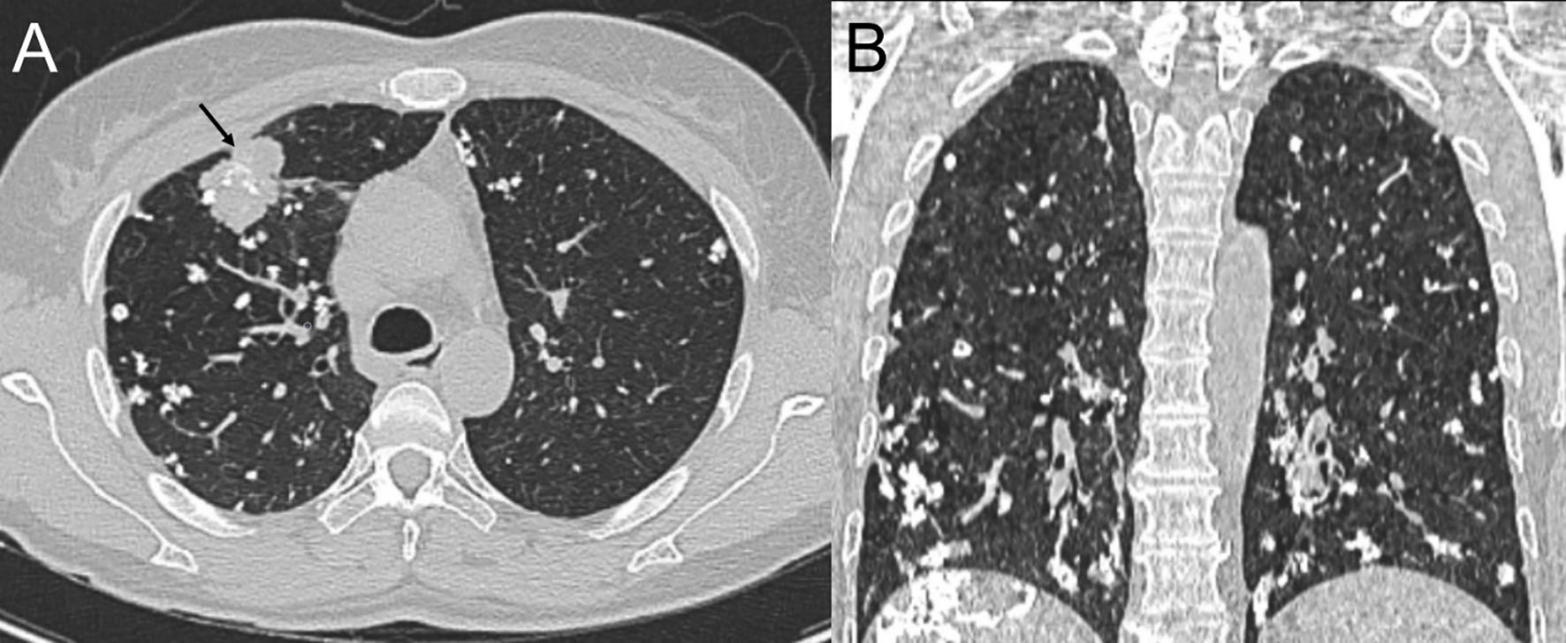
Computed tomography (CT) scan of the chest, lung window, axial view (A) and coronal view (B), showing a mass in the right superior lobe (black arrow) and bilateral soft tissue nodules in the lungs with diffuse calcifications adjacent to the bronchioles and small- and medium-sized vessels.
